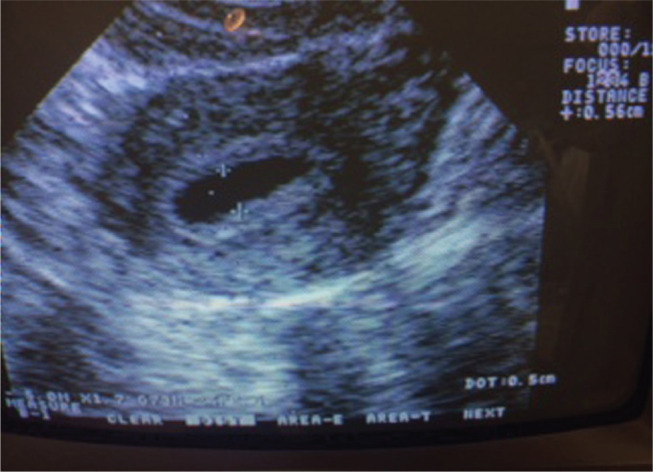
Endometrial cavity fluid (ECF). The day of oocyte retrieval. Fluid accumulation in the uterine cavity detected by transvaginal ultrasound in a sagittal view (A-P diameter 5.6 mm).
